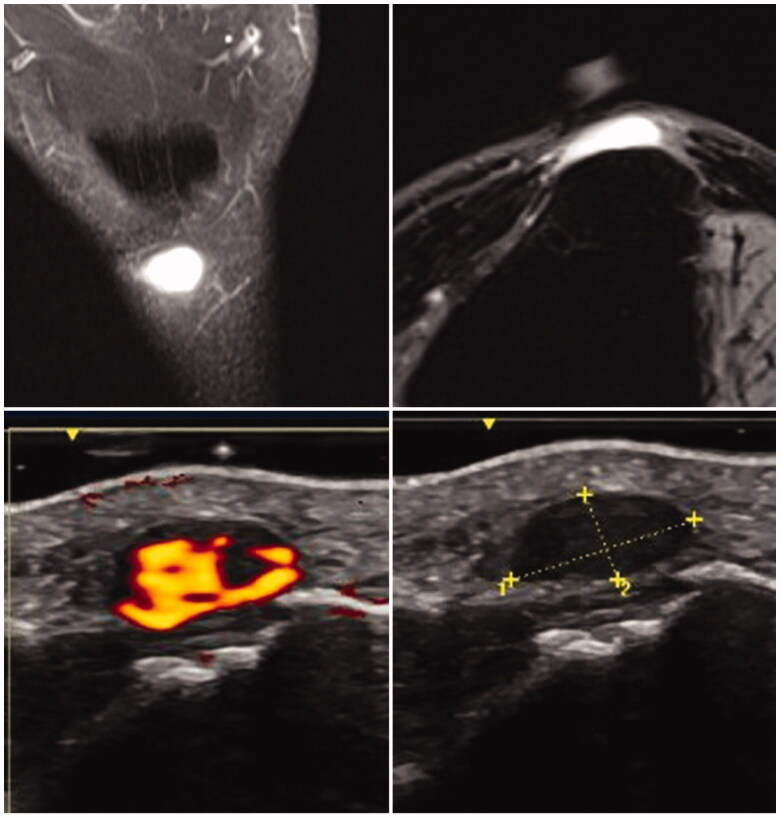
Top images from pre-operative MRI. T1 fat saturated in coronal and axial planes. Bottom images from pre-operative doppler ultrasound.
